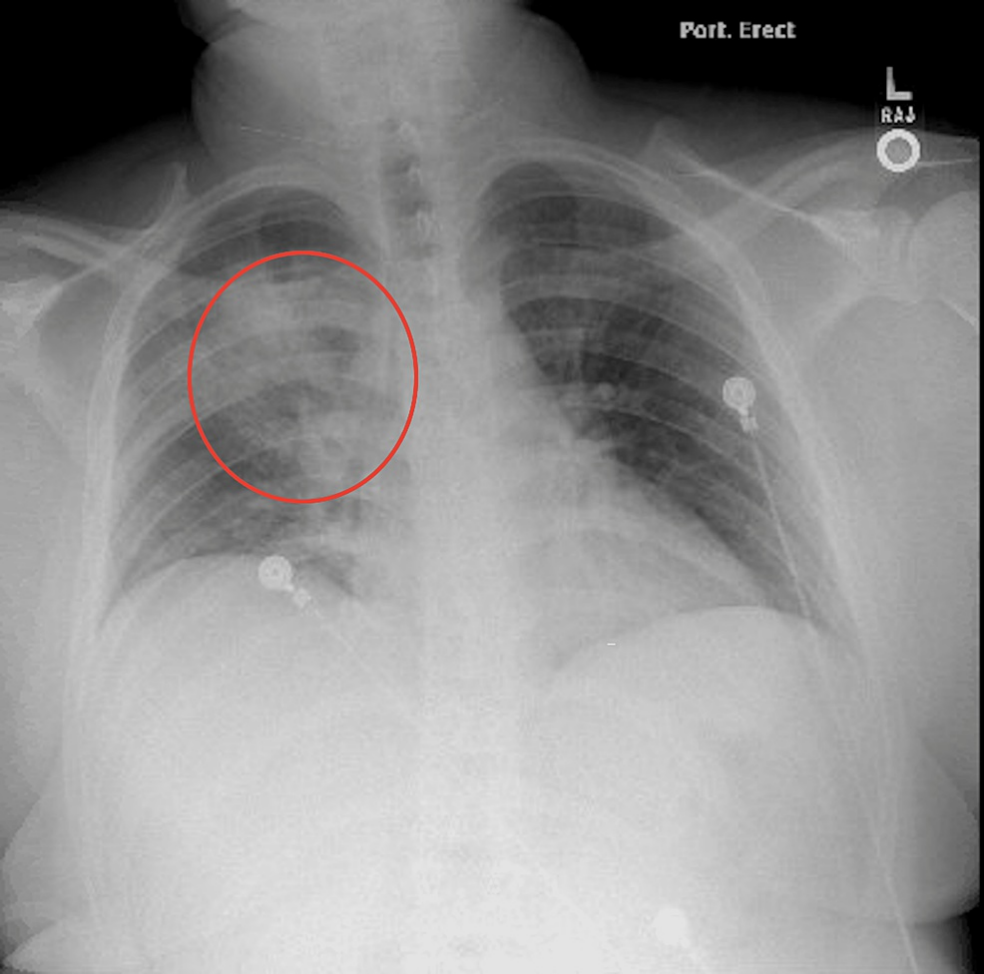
Chest X-ray showing multiple opacities in the right lung
